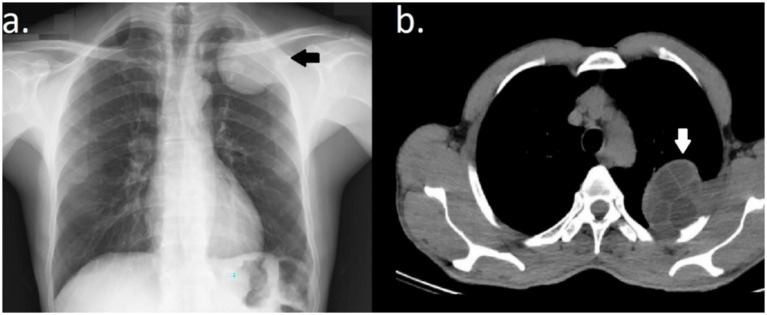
(a) Chest X-ray reveals a wide-based mass located on the costal pleura in the upper zone of the left lung (black arrow). (b) A multilocular cystic lesion with a thick wall, smooth contours, measuring 56 × 52 mm, and containing a few millimetric calcifications, was detected in the apico-posterior segment of the left lung on thorax CT, causing destruction on the second, third, and fourth costae (white arrow).
